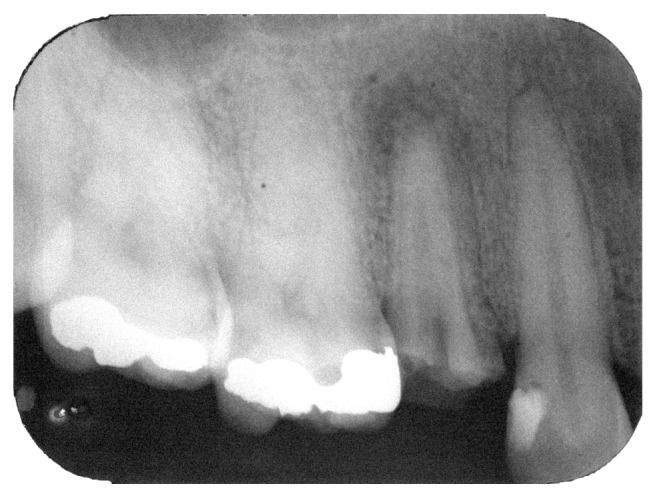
Periapical radiograph confirming the complete removal of the fragment.
